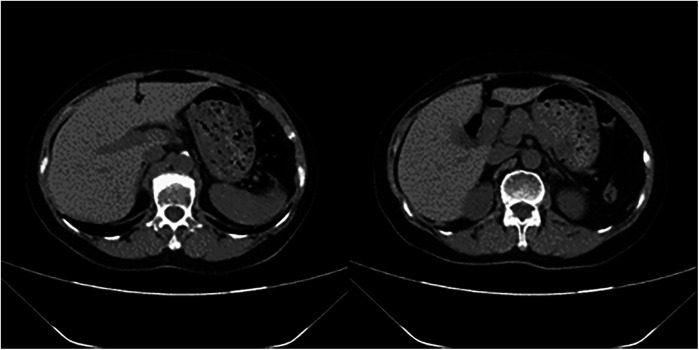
Abdominal CT before discharge.
